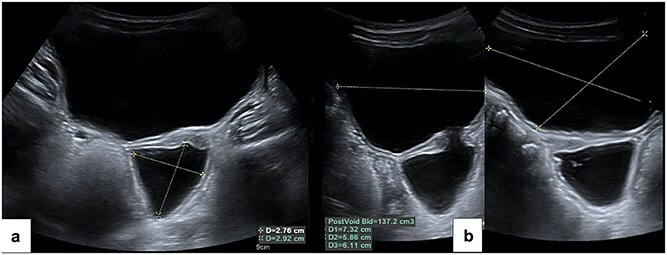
Ultrasound of the urinary tract demonstrating a thin-walled urinary bladder with a pre-void volume of 272 ml (a) and a significant post-void residual volume of 137 ml (b). A cystic structure consistent with a bladder diverticulum is identified arising from the left posteroinferior aspect of the bladder, measuring 29 × 27 mm, with associated urothelial thickening. Colour Doppler demonstrates urinary jets passing from the diverticulum into the bladder lumen.
